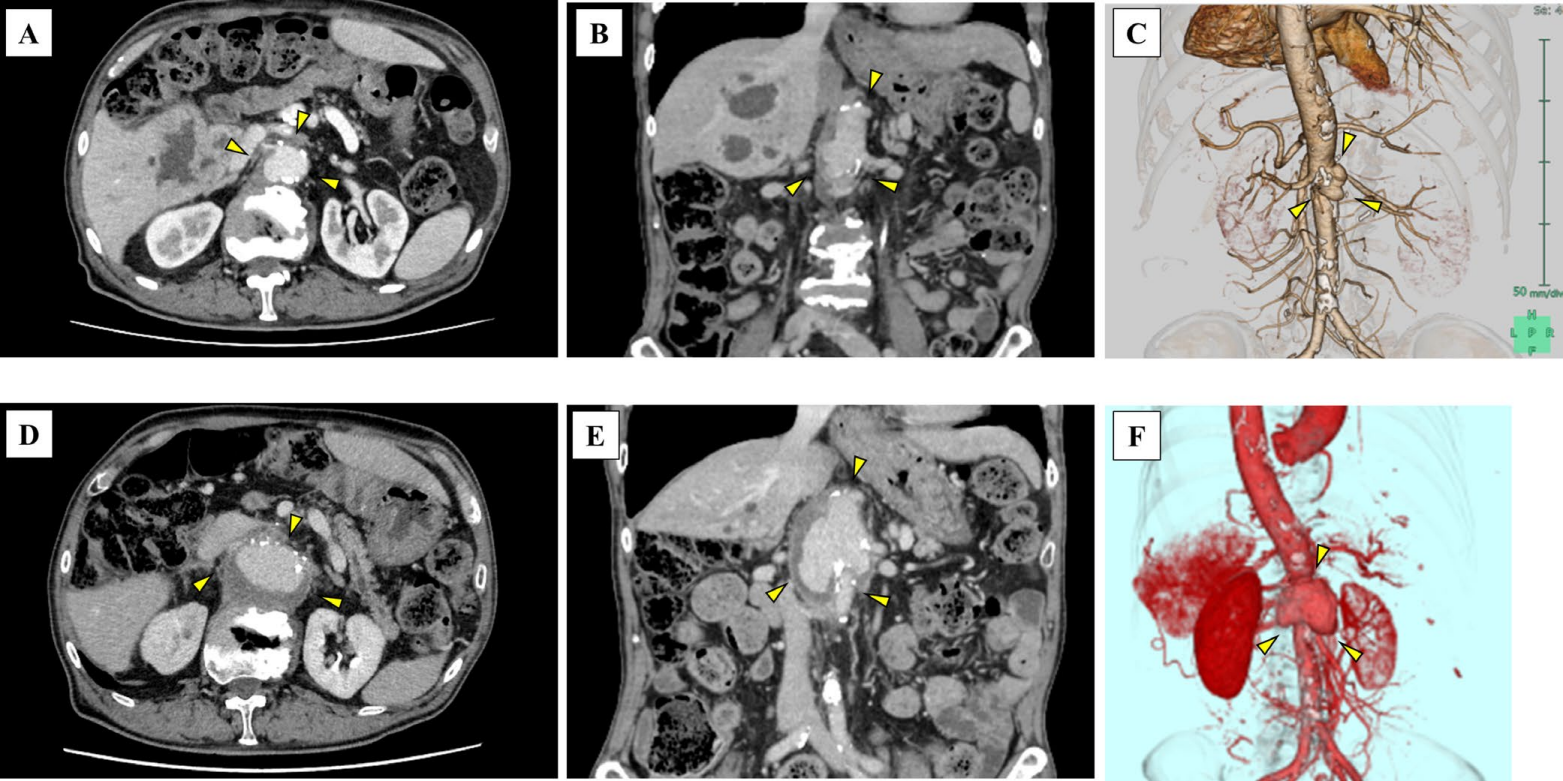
Contrast‐enhanced computed tomography (CT) showing a developing infected aortic aneurysm. (A–C) Contrast‐enhanced CT showing polycystic liver abscesses and an infected aortic aneurysm at 41 mm in maximum diameter (the arrowheads). (D–F) Contrast‐enhanced CT showing an infected aortic aneurysm at 60 mm in maximum diameter (the arrowheads).
